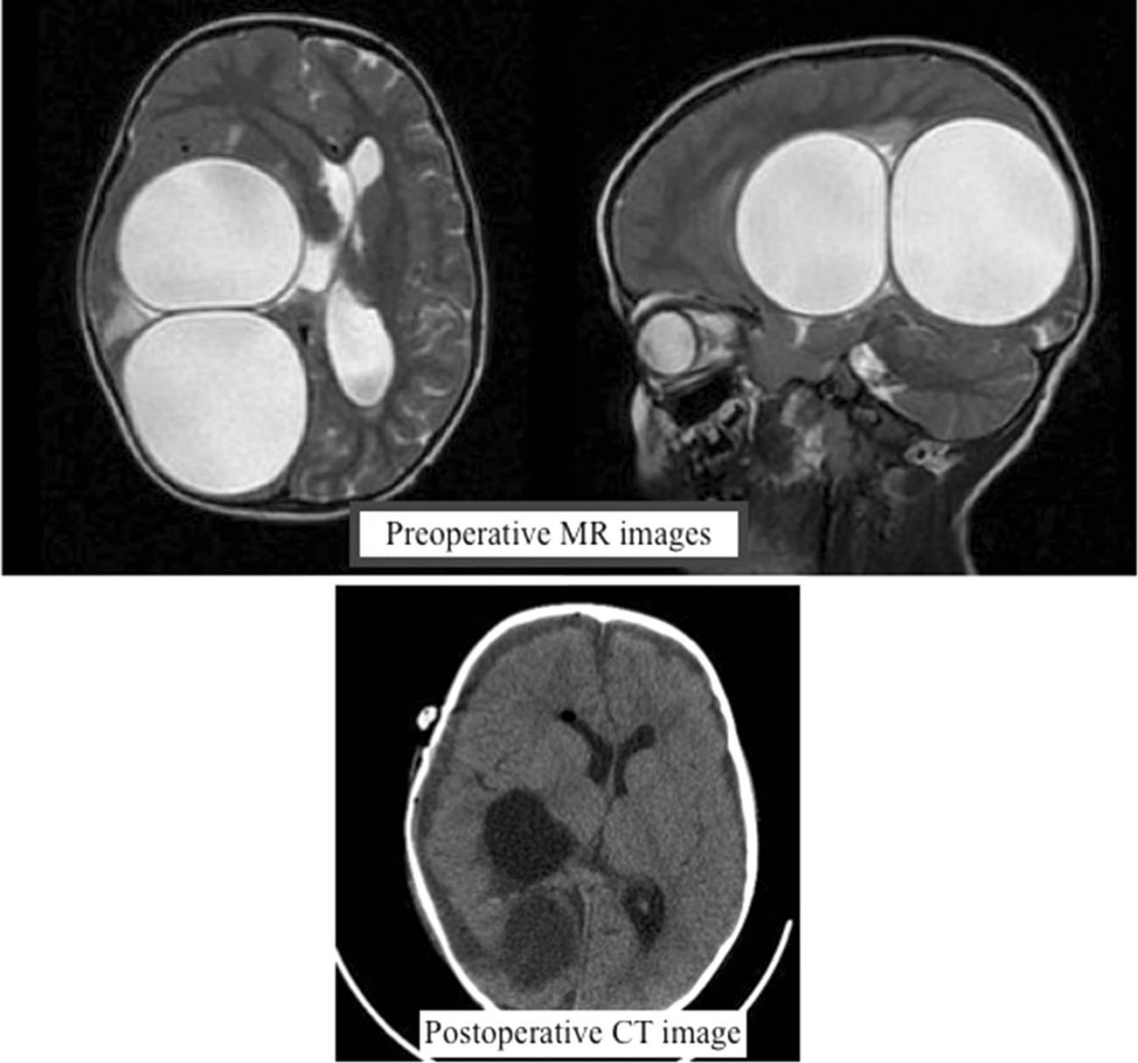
T2-weighted MR image of a smooth-edged, 6-cm diameter hydatid cyst in the right temporal-parietal lobe, with a second smooth-edged 7-cm diameter hydatid cyst starting in the right parietal lobe nearly filling the occipital lobe, anterior and neighbouring the first, exceeding the occipital cortex to the posterior. Cranial CT check after removal of both hydatid cysts, showing reduction in the cyst location and mass effect of the cysts
